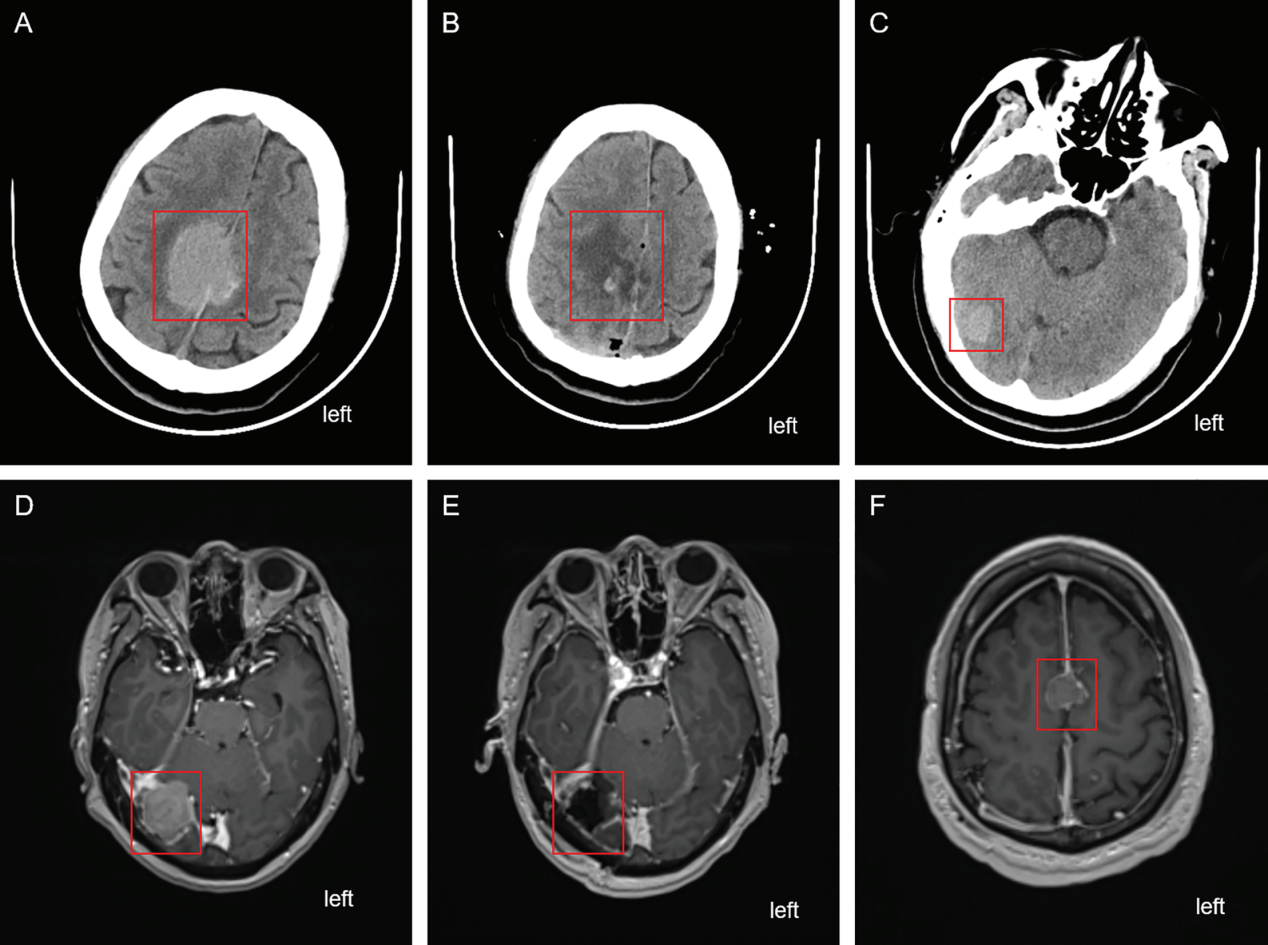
Cranial imaging examination before and after the two brain surgeries. (A) CT scan depicting the falx cerebri tumor before the first brain surgery. (B) CT depicting the falx cerebri region after the first brain surgery. (C) Unresected tumor in the right occipital region after the first brain surgery. (D) Enhanced MRI revealing the enlarged right occipital tumor before the second brain surgery. (E) Enhanced MRI depicting the right occipital region post the second brain surgery. (F) Recurrent and unresected tumor in the frontal lobe region. The red box highlights the location of the tumor lesion
